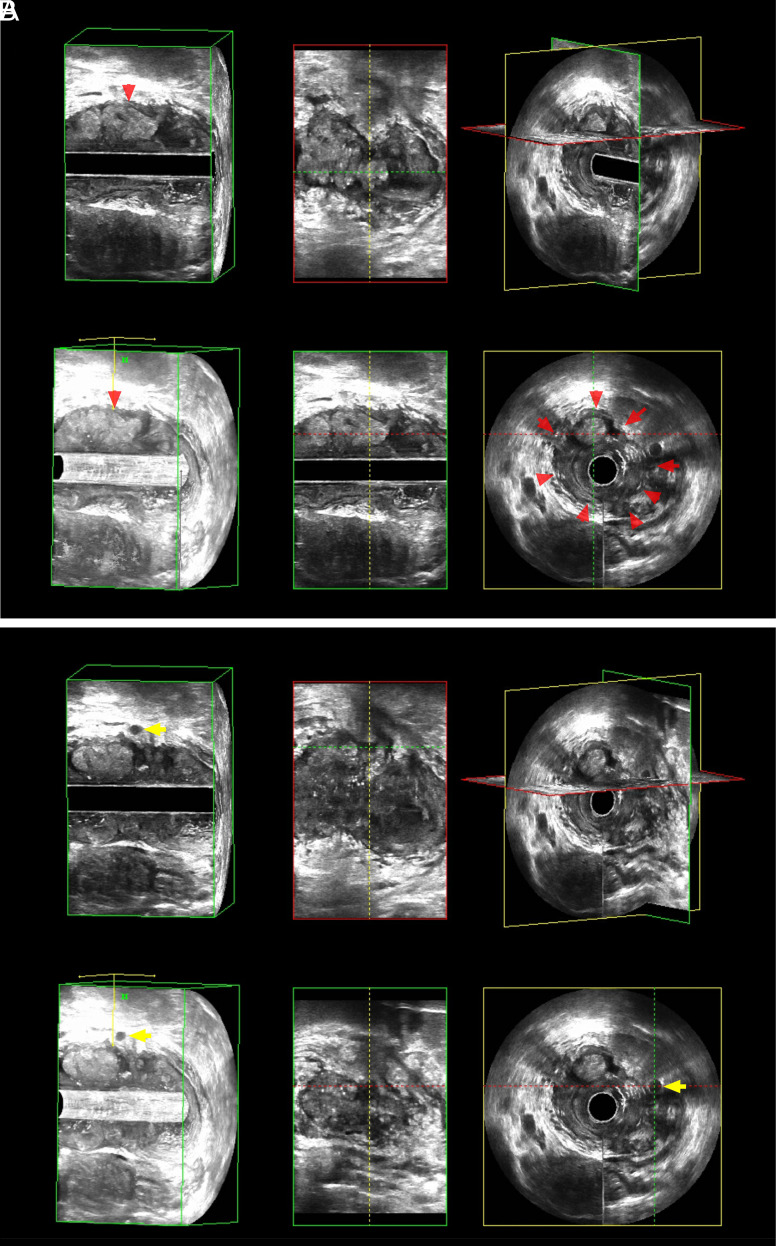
Patient with RC was pathologically confirmed as a representative case of positive LNM. The above-mentioned images are 3D-ERUS scan images, which are 3-dimensional, perspective, sagittal, coronal, cross-sectional, and 3-dimensional sectional views. The red arrow in (A) shows the area of the rectal tumor, and the yellow arrow in (B) shows the lymph nodes around the rectum. 3D-ERUS, 3-dimensional endoanal rectal ultrasound; LNM, lymph node metastasis; RC, rectal cancer.
